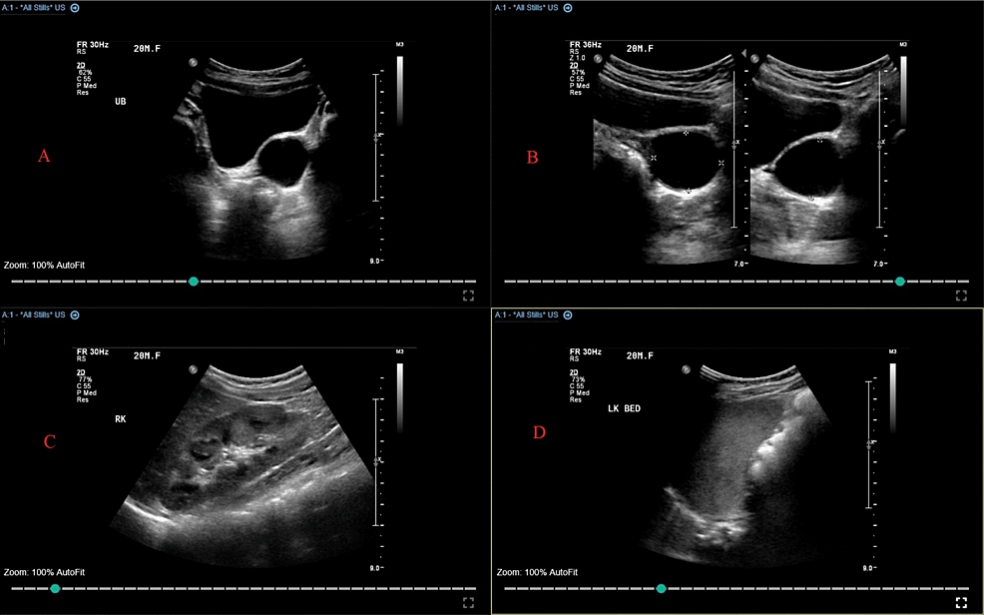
Renal ultrasonography scan showing pelvic cystic lesion posterior to the bladder (A, B), normal right kidney (C), and absent left kidney (D)
